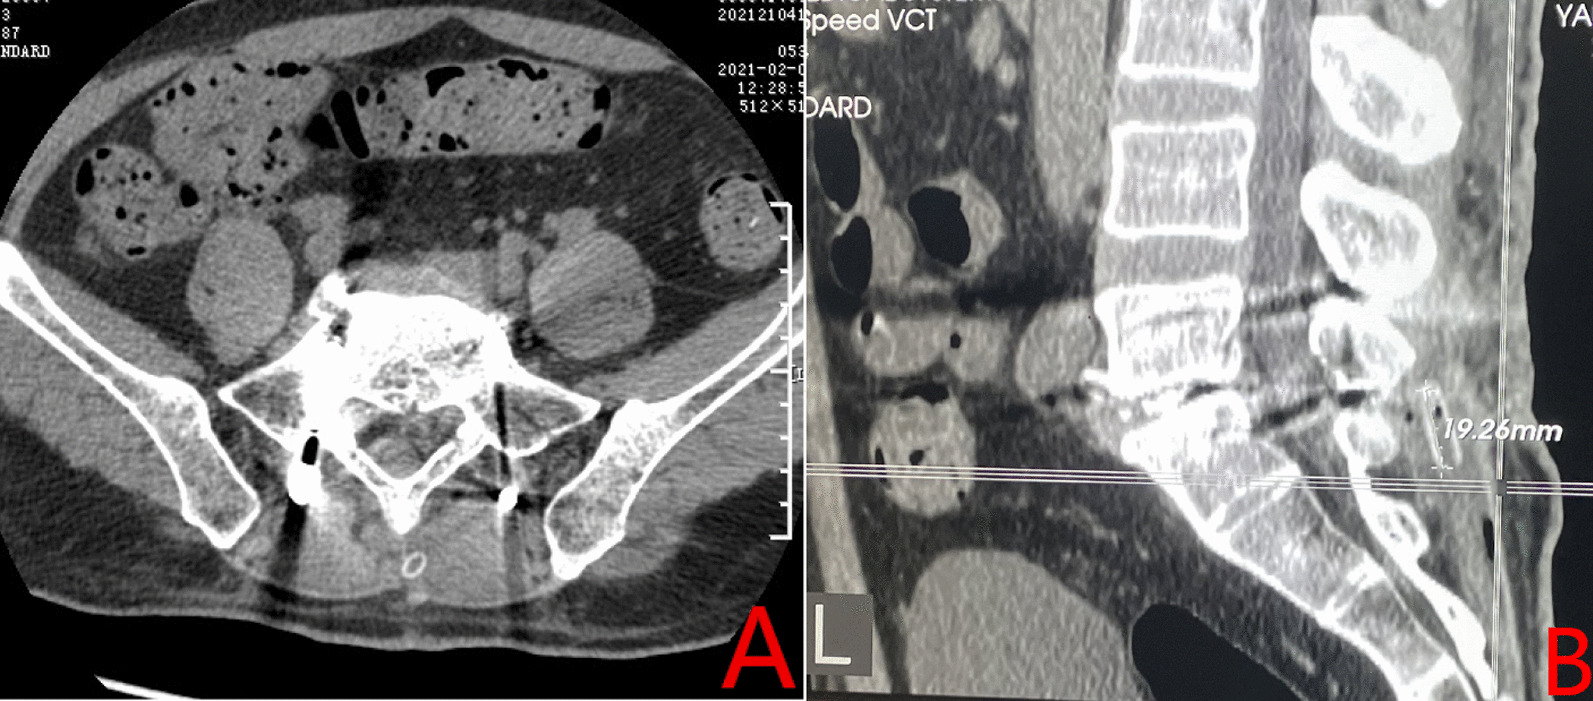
Postoperative computed tomography (CT) scans. A Postoperative CT scan. B Postoperative CT sagittal scan taken on the 3rd postoperative day. The 2 cm long broken drainage tube can be seen behind the spinous process
